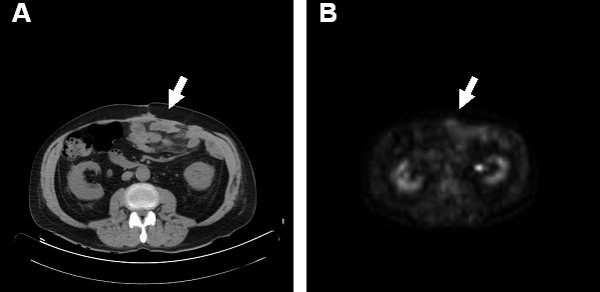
Imaging studies after RA treatment with etanercept and prednisone. (A) CT exam of the abdomen demonstrating moderate resolution of the previous mass/lesion shown in Figure 2 Panel A (arrow). (B) FDG-PET transverse image demonstrating decreased tracer uptake in the anterior abdominal lesion after treatment of RA (arrow).
